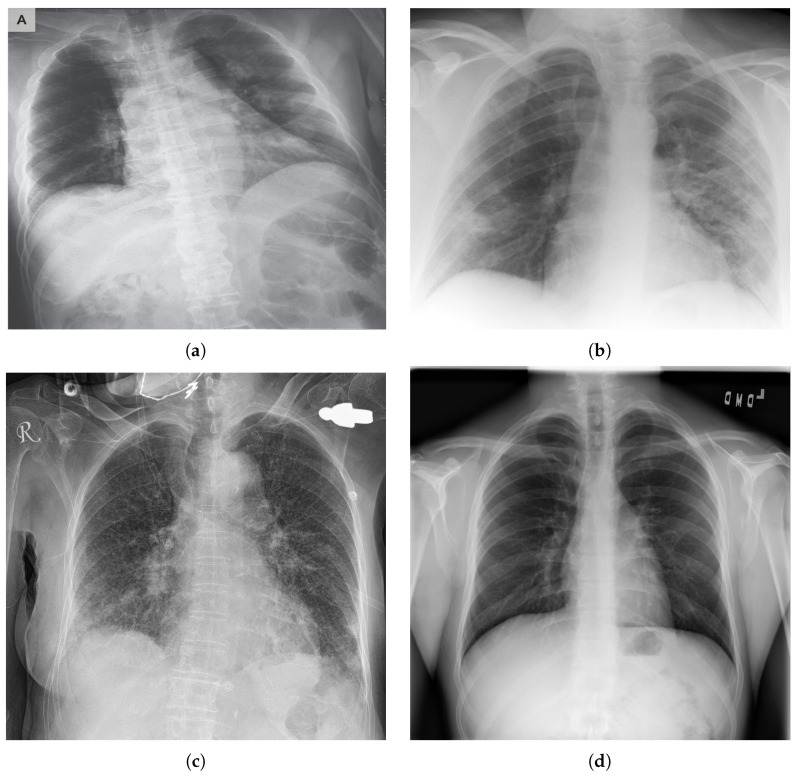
Chest X-ray of a person (a) with a COVID-19, (b) with COVID-19 pneumonia, (c) with pneumonia, and (d) a normal case.
